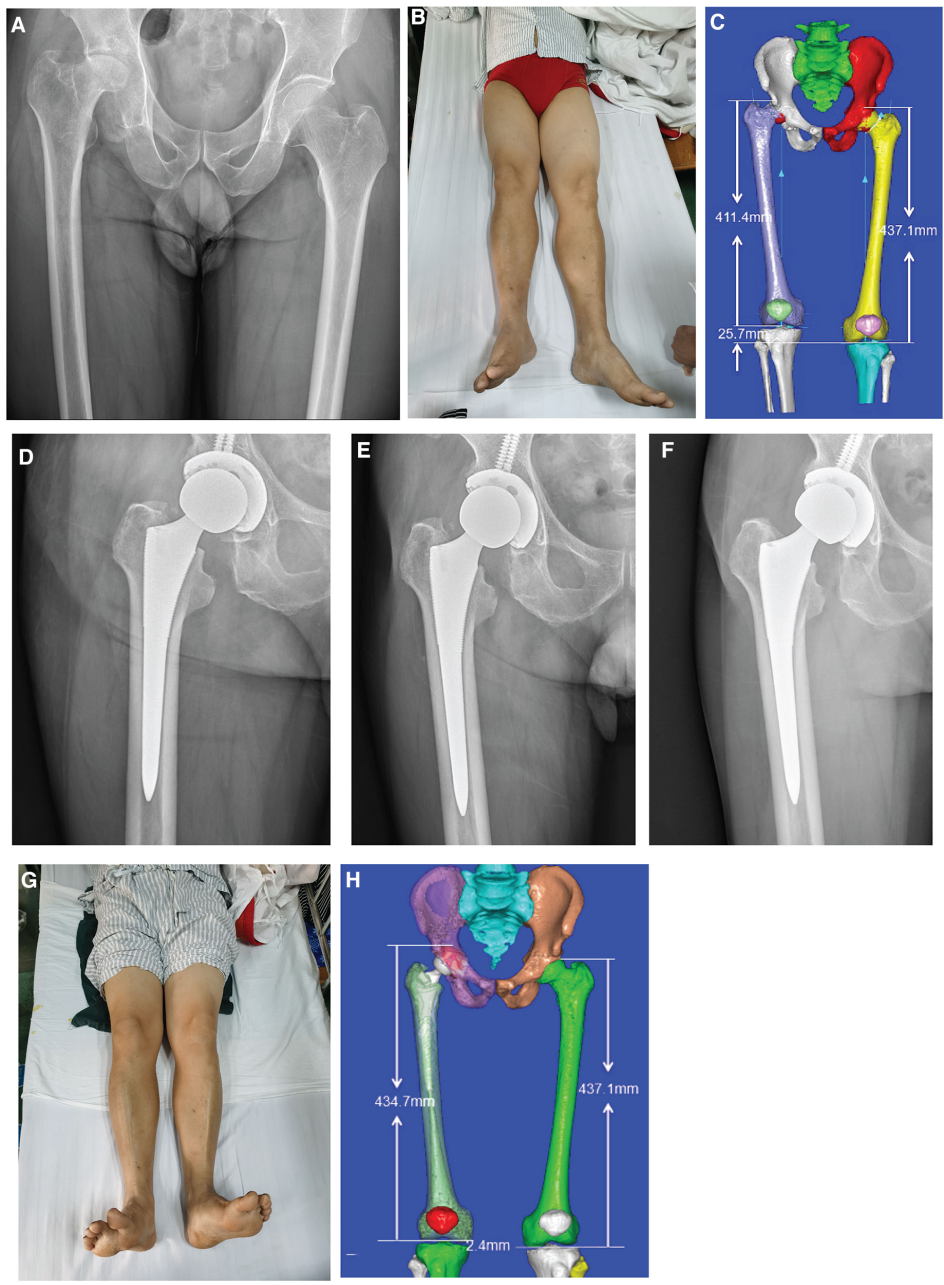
Typical case of CAD preoperative planning: (A) preoperative pelvic x-ray showing osteonecrosis of the femoral head (right side); (B) preoperative appearance of both lower limbs showing an obvious shortening of the right lower limb; (C) preoperative three-dimensional measurement of lower limb length difference; (D–F) follow-up radiographs at 1, 3, and 6 months postoperatively; (G) postoperative appearance of lower limbs; and (H) postoperative three-dimensional measurement of the lower limb length difference.
